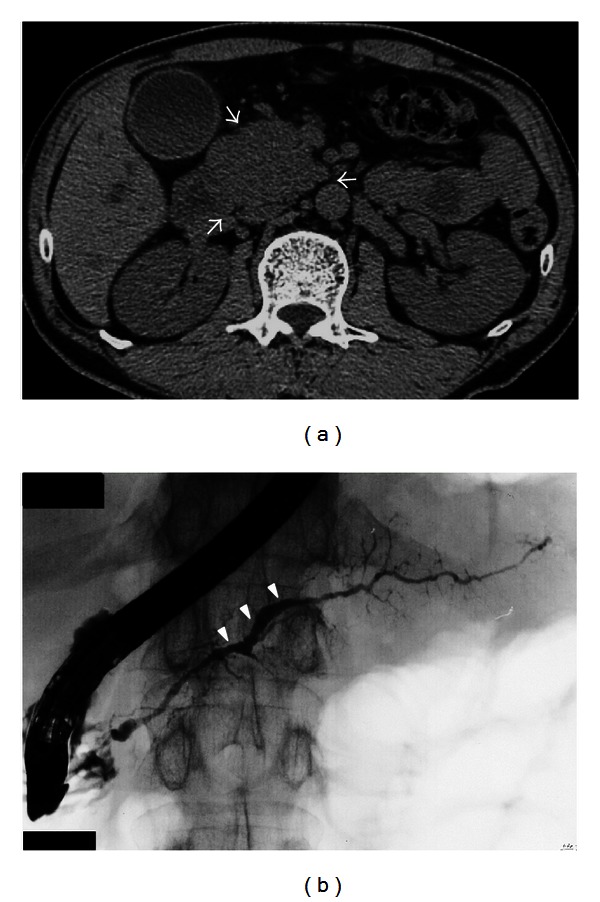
CT and ERCP findings of AIP showing independent risk factors for progression to confirmed chronic pancreatitis at diagnosis. (a) Pancreatic head swelling (arrows). (b) MPD nonnarrowing in the pancreatic body (arrowheads).
